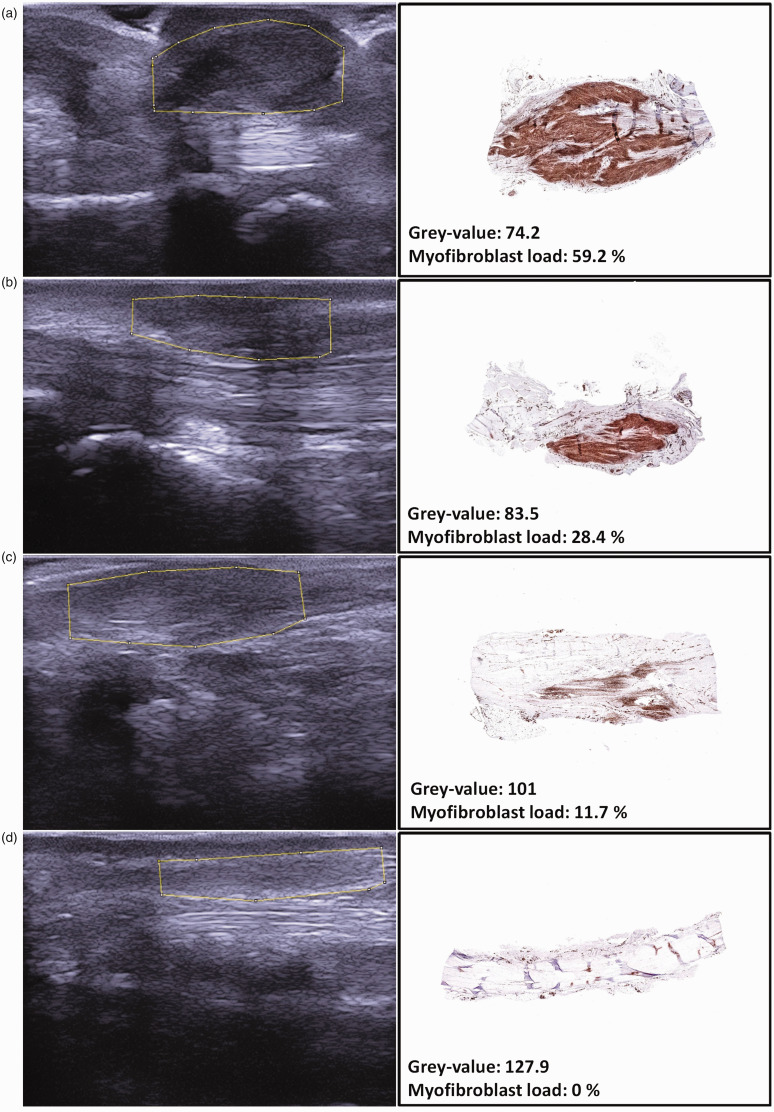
Example of nodules with different grey-values and myofibroblast loads. Subjectively, nodules become lighter on ultrasound from image (a) (darkest) to (d) (lightest), which is objectified by an increasing mean grey-value, measured with Image J. As the grey-value increases, the myofibroblast load decreases.
